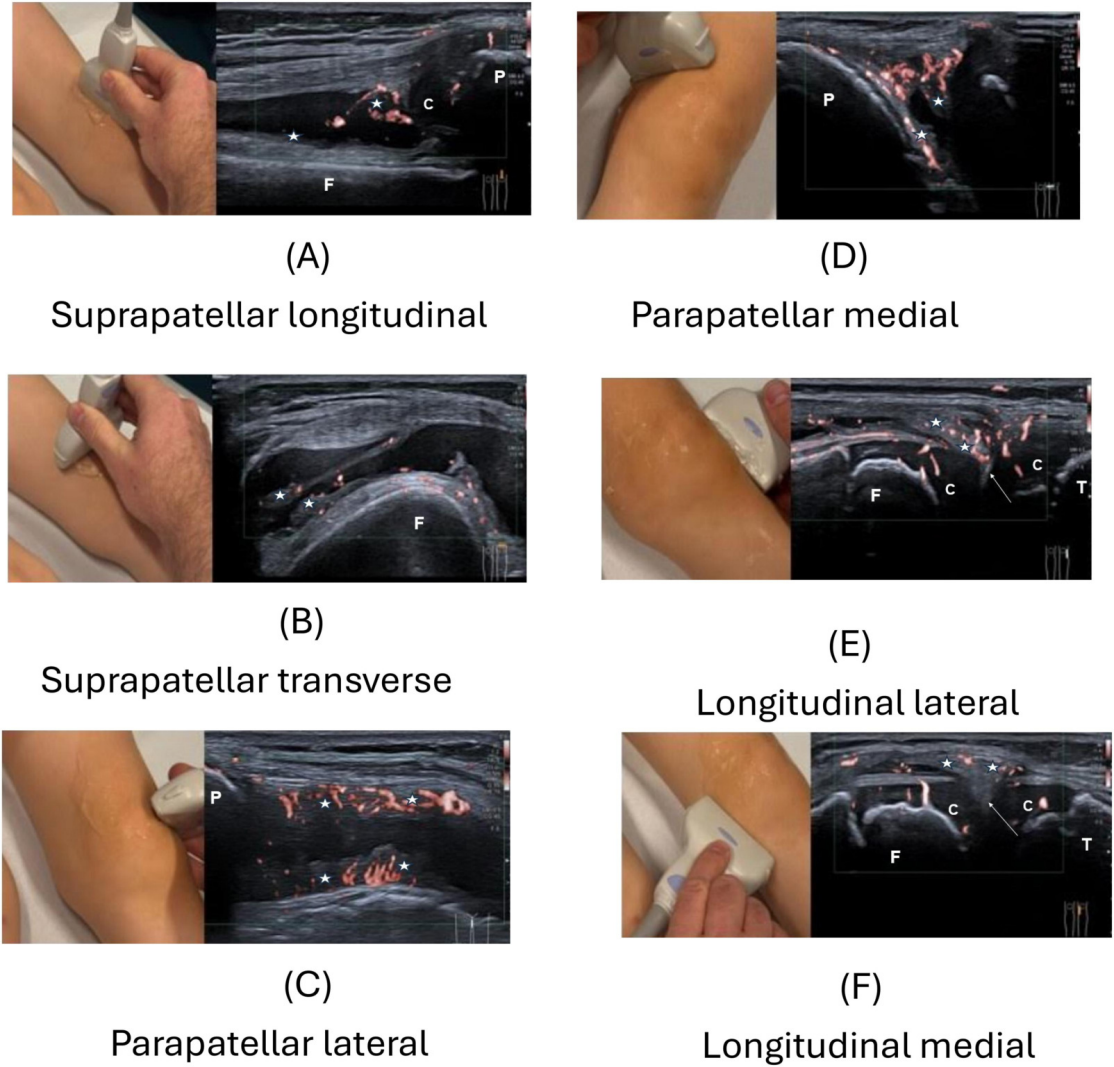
All six standard scanning positions for ultrasound (US) of the knee according to pediatric OMERACT are shown, on the right the respective US image, on the left the corresponding probe position: (A) suprapatellar longitudinal scan, (B) suprapatellar transverse scan, (C) parapatellar lateral transverse scan, (D) parapatellar medial transverse scan, (E) longitudinal lateral scan, and (F) longitudinal medial scan. C, cartilage; P, patella; F, femur; T, tibia; star, synovia; arrow, meniscus.
